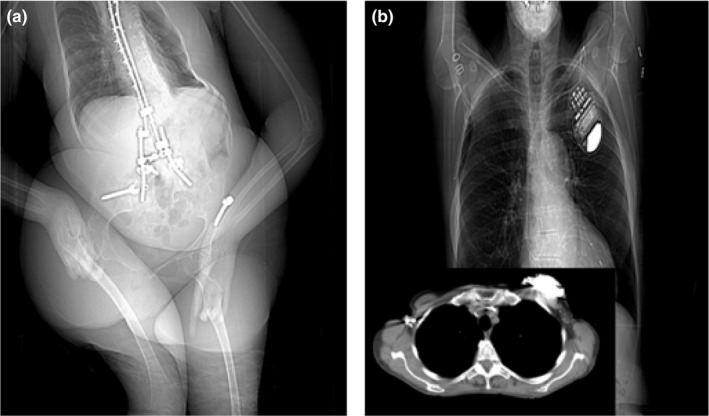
Other examples of how ACR DIR threshold algorithm causes an outlier case. Shown here are CT localizers where (a) the arms down and (b) a device is resting on a patient that will only be included in the CT axial scan extraction of patient geometric size surrogates.
